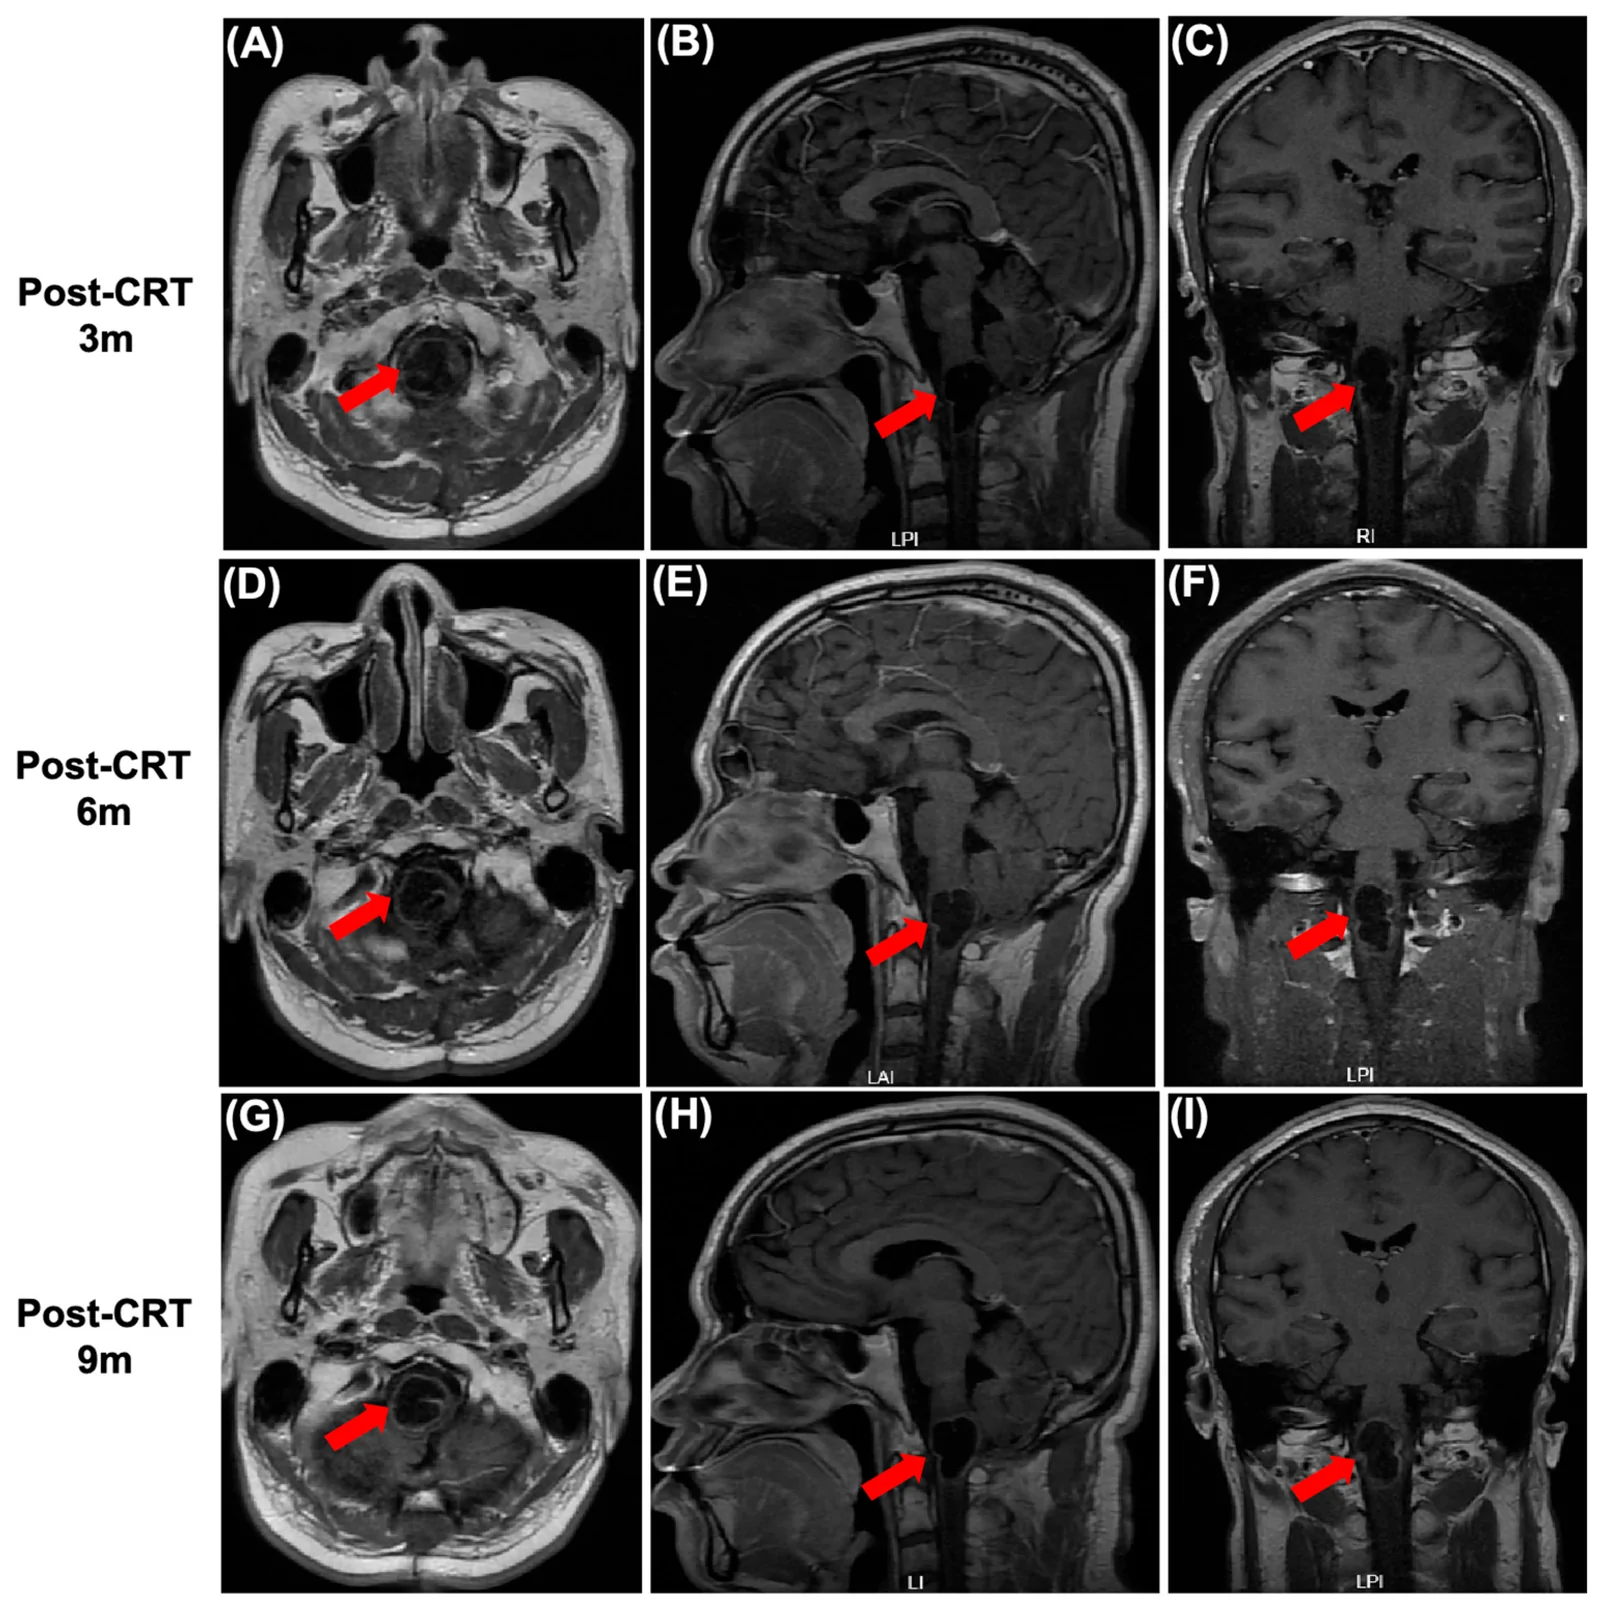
Cranial MRI images showing brainstem lesions at different time points after CRT. (A–C) Axial, sagittal, and coronal views of cranial MRI show a tumor mass measuring 20 mm × 19 mm × 33 mm 3 months after CRT; (D–F) axial, sagittal, and coronal views of cranial MRI show a tumor mass measuring 20 mm × 17 mm × 33 mm 6 months after CRT; and (G–I) axial, sagittal, and coronal views of cranial MRI show a tumor mass measuring 20 mm × 17 mm × 33 mm 9 months after CRT. The position pointed by the arrow is the site of the lesion. On 11 April 2024, he visited the clinic again 9 months after CRT, reporting that the slight worsening of right limb weakness he had experienced had persisted for nearly 2 weeks. He then received the first cycle of chemotherapy with 150 mg/m^2^ TMZ 5/23, followed by five additional cycles of 200 mg/m^2^ TMZ 5/23. After completing six cycles of TMZ chemotherapy, a significant reduction in tumor size was observed (Figure 6A–C). Subsequently, the patient underwent 12 cycles of TMZ maintenance chemotherapy. The last follow-up cranial MRI was conducted in February 2026, and the therapeutic effect was classified as continuous partial remission (PR), with a tumor mass measuring 12 mm × 11 mm × 11 mm (Figure 6D–L). His symptoms improved slightly, with only mild limping remaining, and no serious radiotherapy complications were observed during the treatment period or throughout subsequent follow-up.
